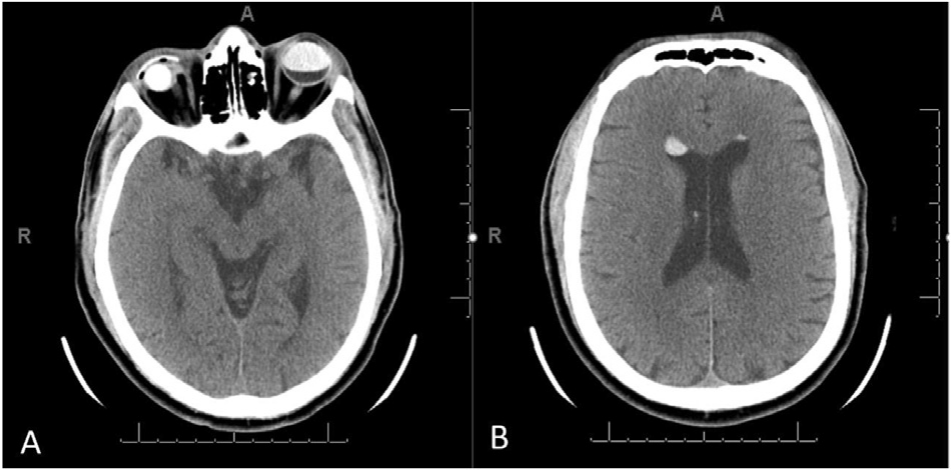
(A) Axial CT scan of the head at the level of the eyes showing changes from a right eye evisceration and acrylic conformer insertion in the past and an ovoid hyperdensity in the left globe that corresponds to intraocular silicone oil, which was used to endotamponade a previous retinal detachment. (B) Axial CT scan of the head at the level of the lateral ventricles showing 2 ovoid hyperdensities in the frontal horns bilaterally. The hyperdensities are located anteriorly, because the patient is supine, and the silicone oil has lower density than the cerebrospinal fluid.
